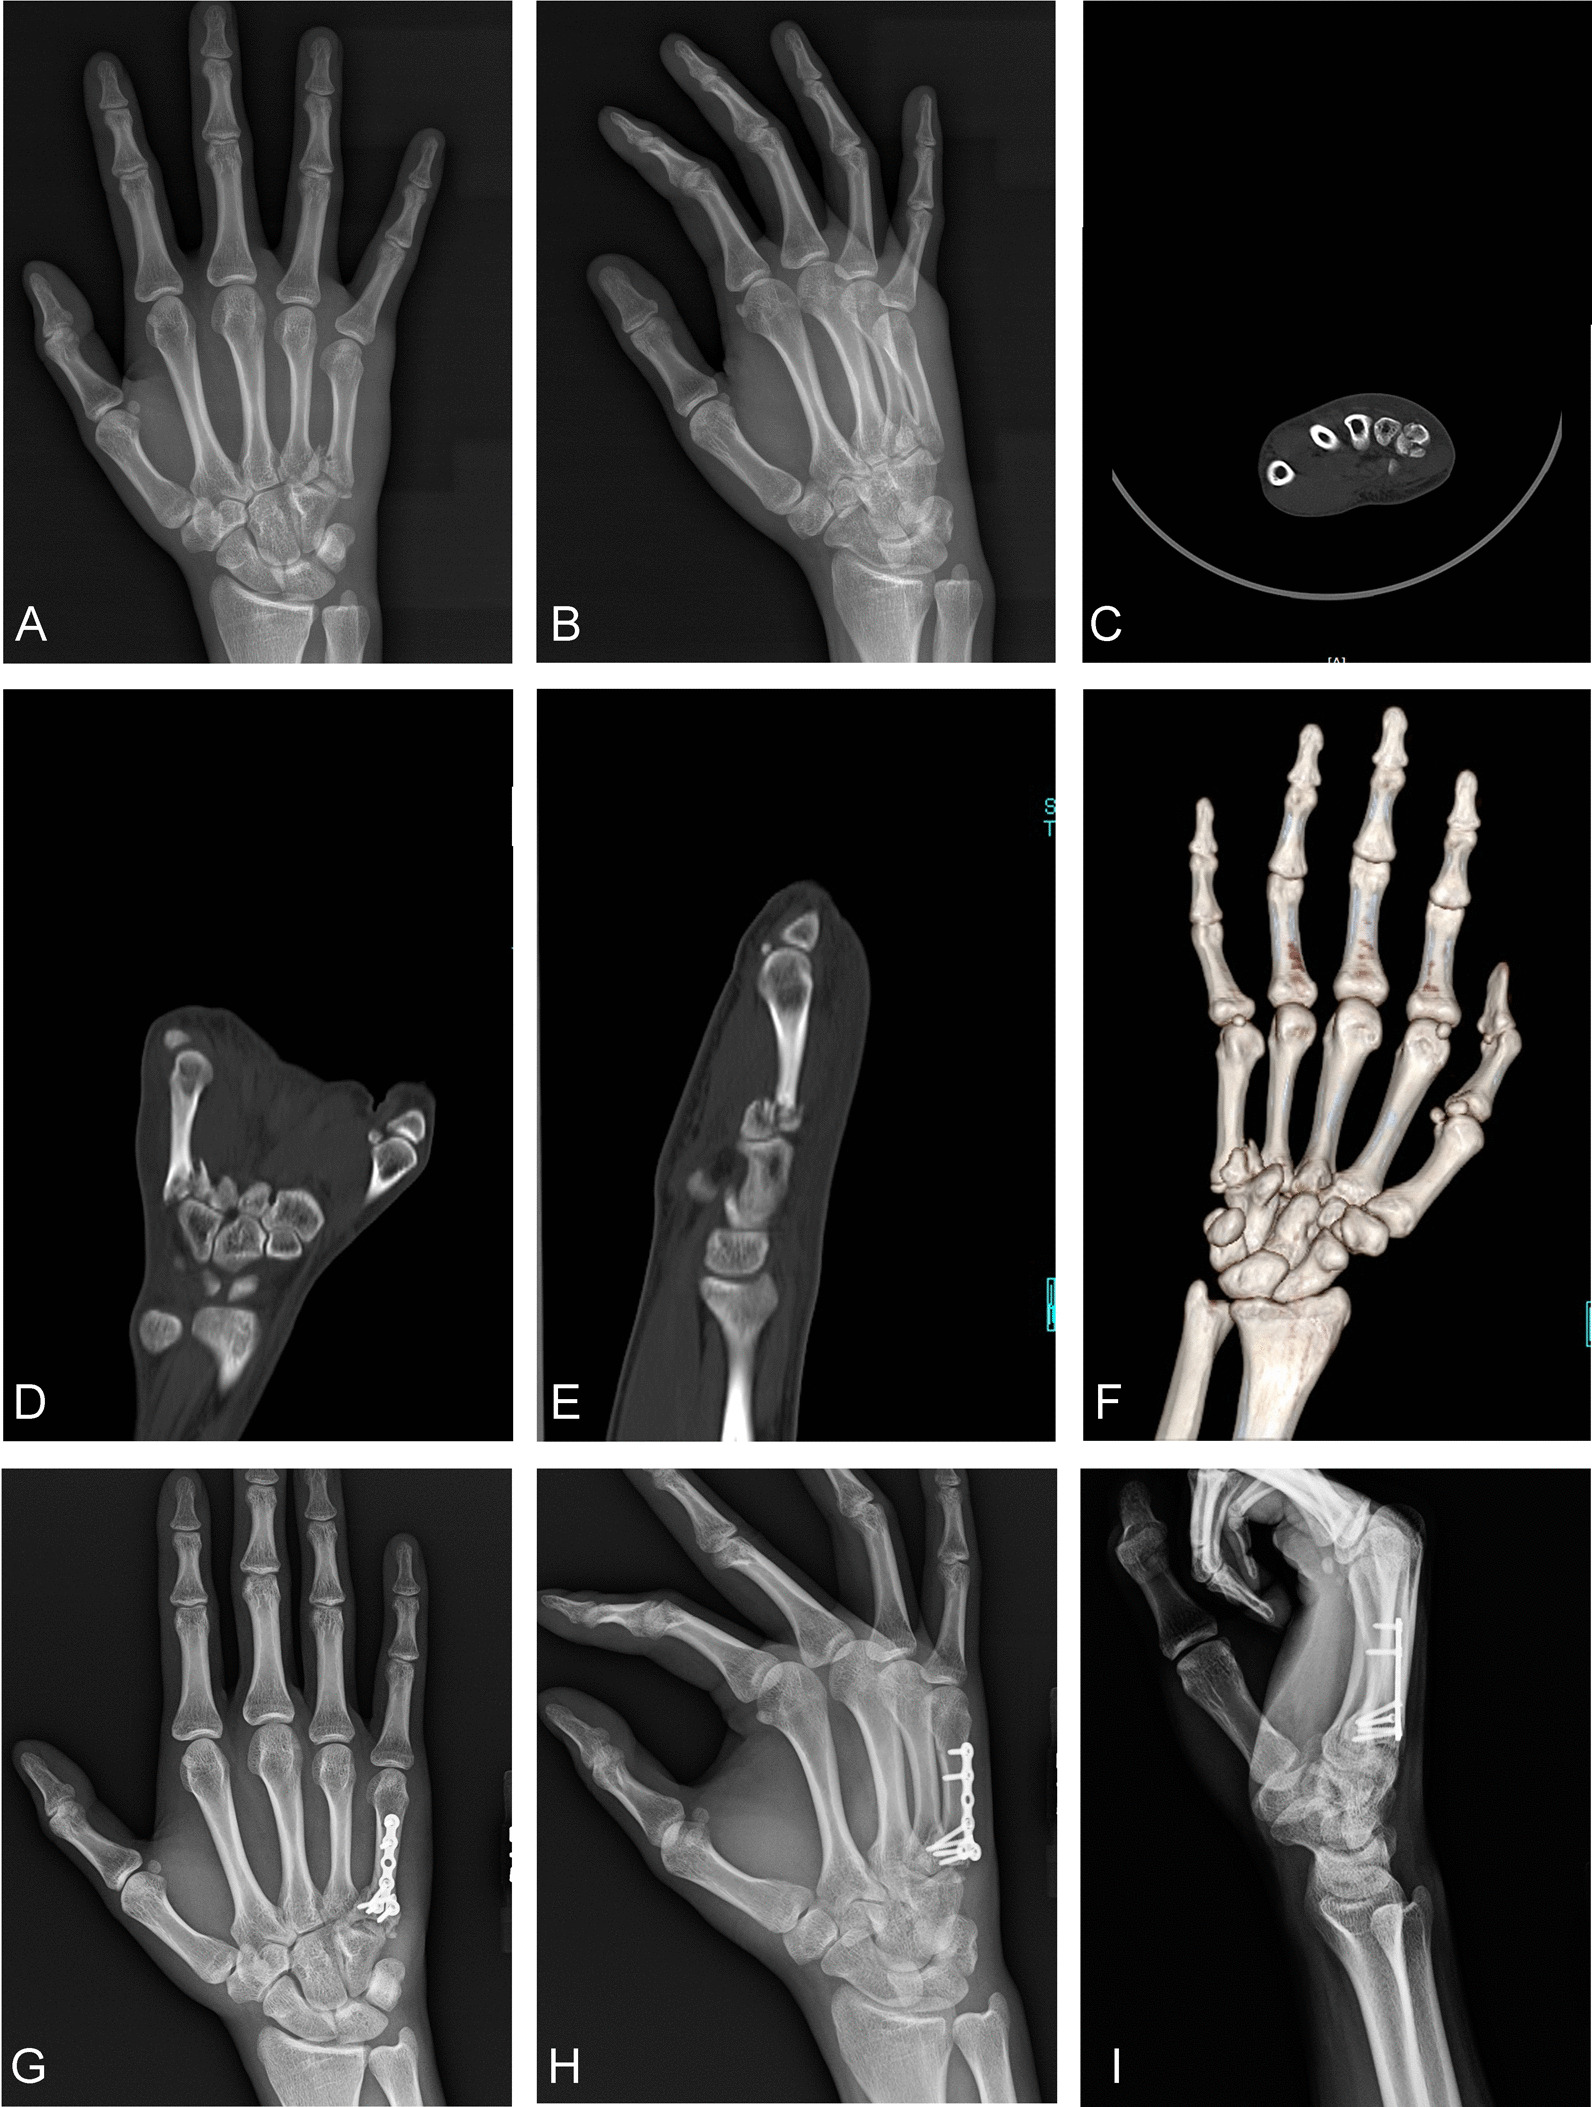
A, B The preoperative posteroanterior and lateral oblique views of the affected hand on radiograph indicate that there are comminuted fractures of the base of the fifth metacarpal bone, complicated with dislocation. C, D, E, and F Preoperative computed tomography and three-dimensional reconstruction of the affected hand show a comminuted fracture of the base of the fifth metacarpal bone that is complicated with dislocation. G, H, and I One-month postoperative posteroanterior and lateral oblique views on radiograph show that the steel plate was not fixed across the joint, and one screw was used to fix the broken fracture block
